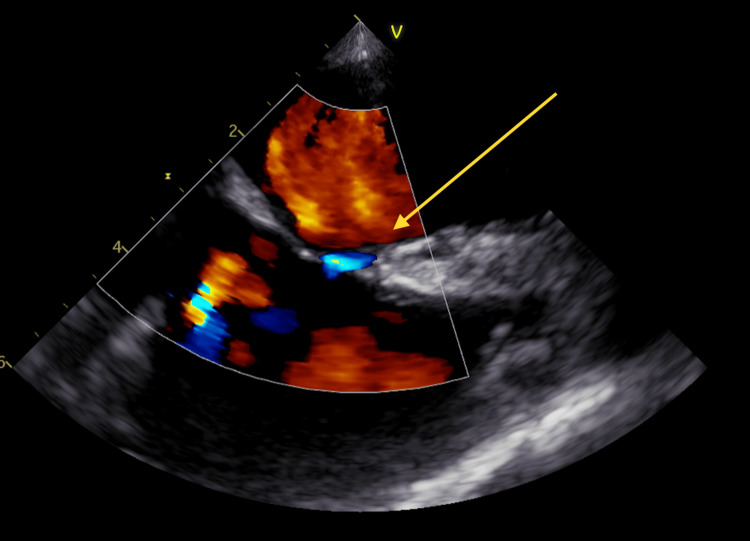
Patent foramen ovale (PFO) demonstrated using color flow Doppler during TEE TEE: trans-thoracic ECHO
